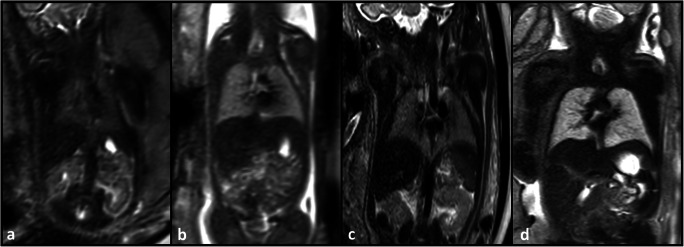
Coronal T2-weighted fetal MR images (a–d) of two fetuses with premature rupture of membranes and subsequent anhydramnios and pulmonary hypoplasia (a, c) at gestation weeks 23 (a) and 32 (c), and of two fetuses with normal lung development (b, d) at gestation weeks 23 (b) and 32 (d). Fetuses with premature rupture of membranes, anhydramnios and pulmonary hypoplasia exhibit hypointense lung tissue (a, c) compared to the lung tissue of normal controls at the same gestational age (b, d). Radiomics analysis can quantify deviations in shape and microstructural tissue qualities and may in the future complement lung volume to improve prenatal assessment of lung development
